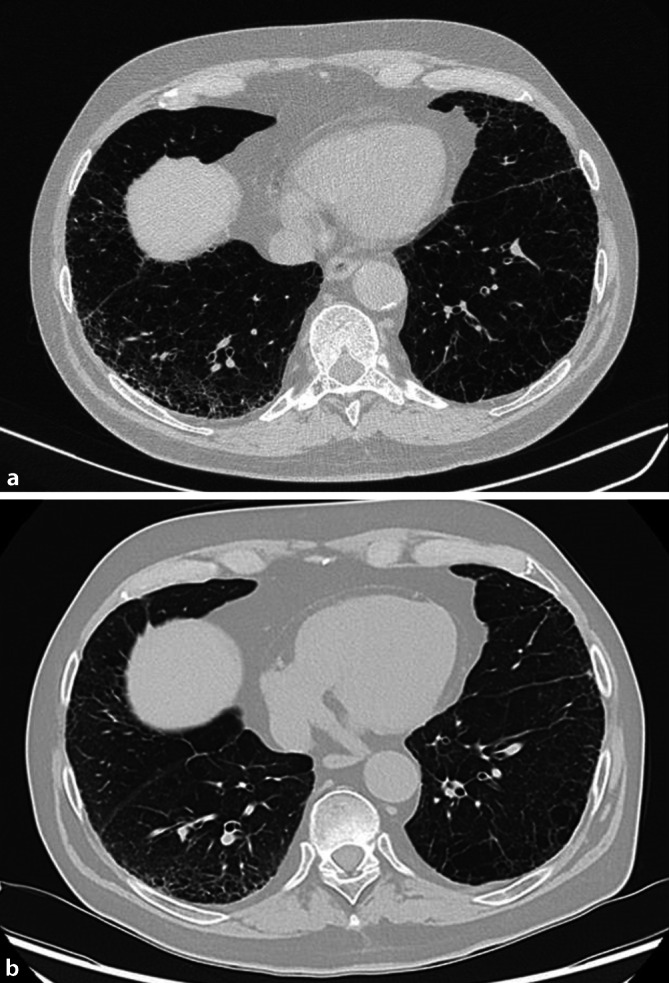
a Baseline: hochauflösende Computertomographie (HRCT) der Lunge mit Nachweis von Milchglastrübungen und Retikulationen. b Monat 6: hochauflösende Computertomographie (HRCT) der Lunge mit Nachweis einer Regredienz der Milchglastrübungen
